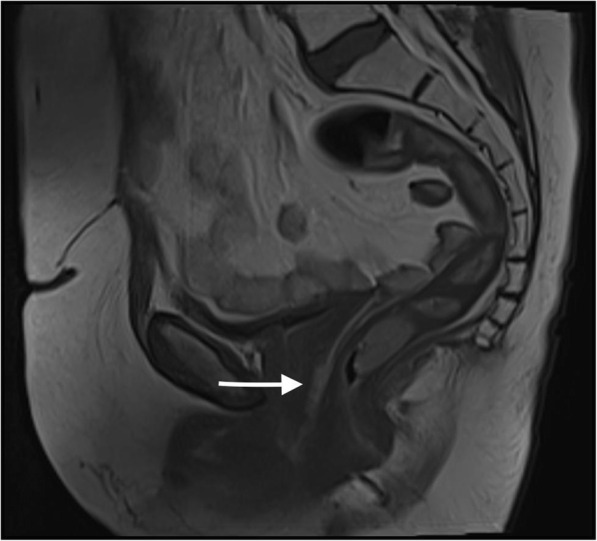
Sagittal T1-weighted image obtained after administration of contrast material demonstrates longitudinal thickening and enhancement of vaginal walls distally (arrow)
